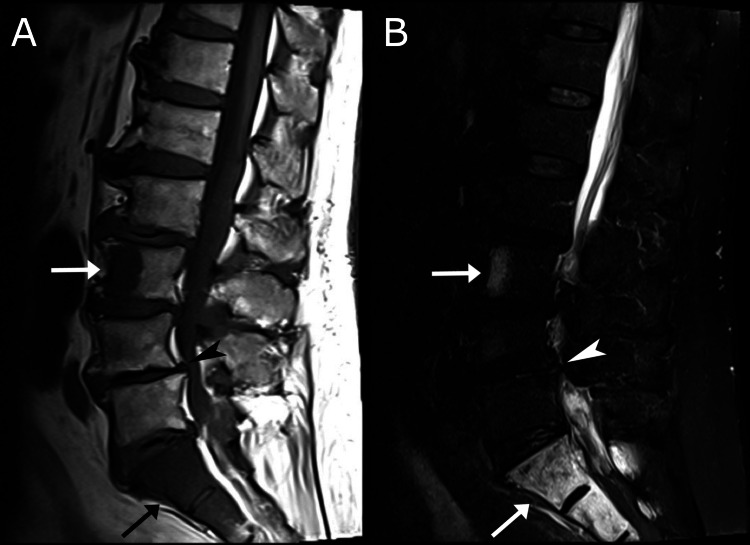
Lumbar magnetic resonance imaging (MRI), T1-weighted (A) and STIR (B) mid-sagittal view. A grade 1 L4/5 degenerative anterolisthesis (arrowhead), and disc-osteophyte complexes at L4/5 and L5/S1 are evident. Marrow replacement (arrows) is evident at the anterior region of L3 and upper sacrum, with a hypointense signal on T1 (A) and hyperintense signal on the STIR image (B), suggestive of metastasis. STIR, short tau inversion recovery
